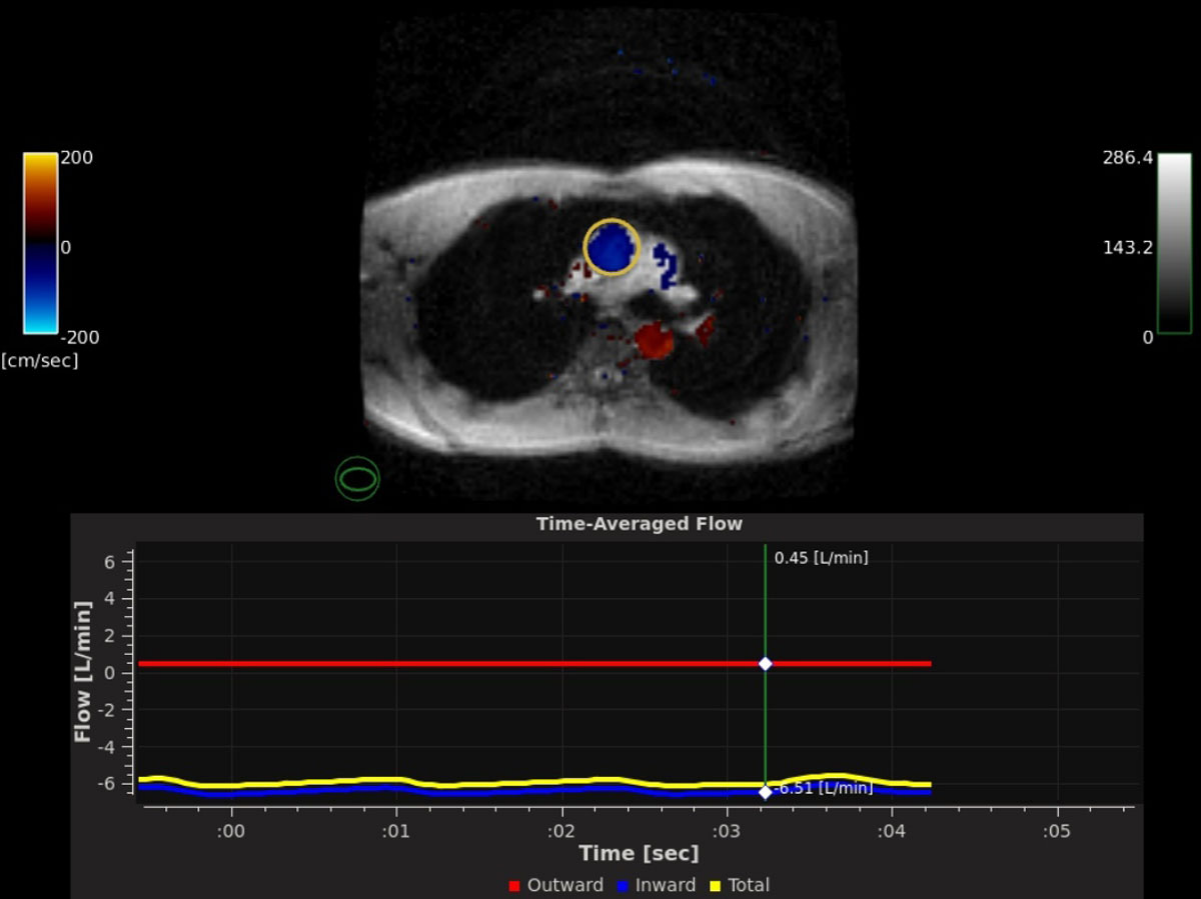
A sample DICOM result from the real-time PC-MRI sequence. The Phase-Contrast data is acquired in real-time and overlaid on GRE magnitude images (top). The time average flow of the phase-contrast data is measured within an ROI (yellow circle) and displayed on a graph in real time (bottom).
